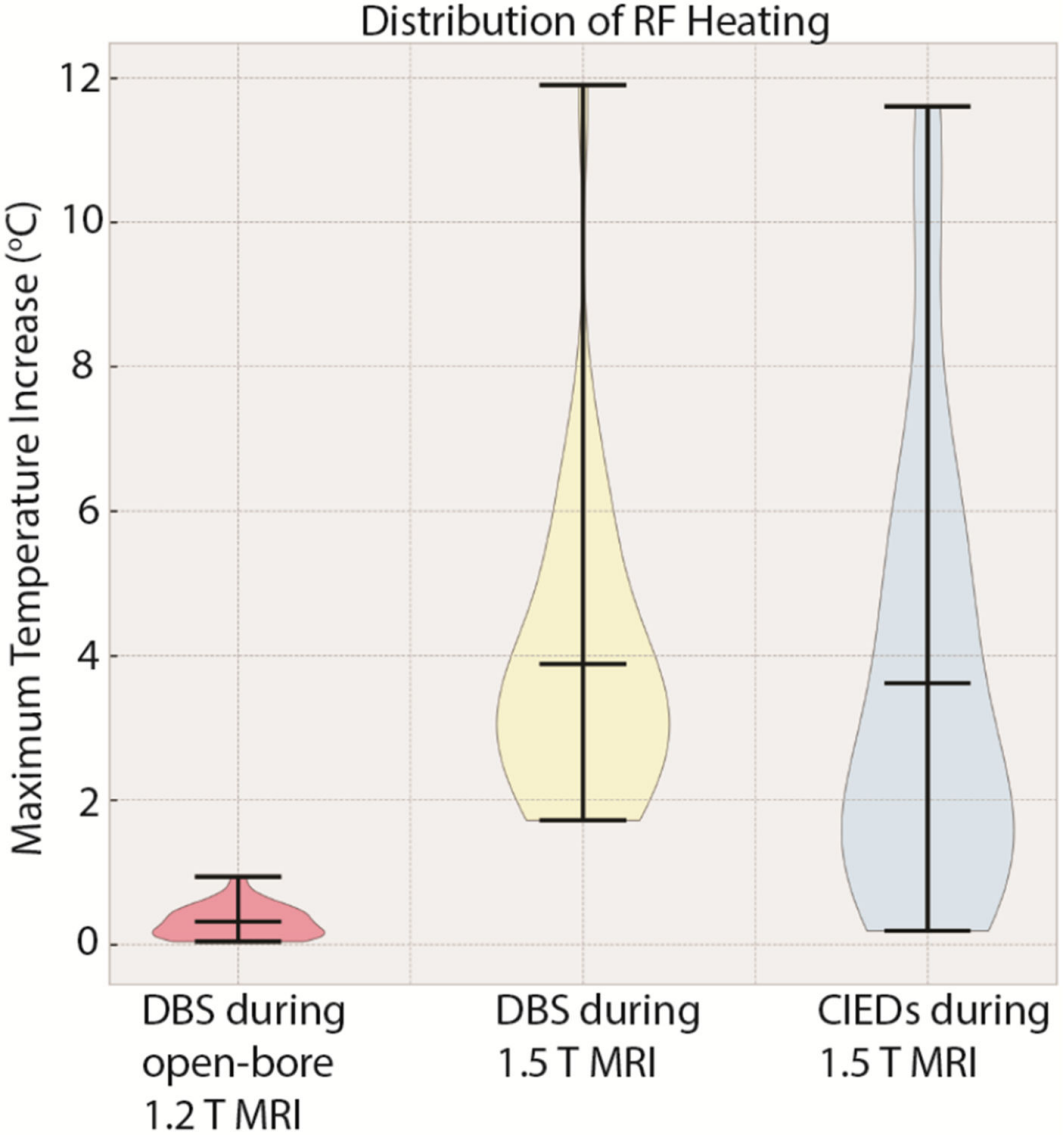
Distribution of ΔT_max_ for cases in the additional test datasets including RF heating of a DBS system during MRI in an open-bore scanner and MRI at 1.5 T and of CIEDs during MRI at 1.5 T.
